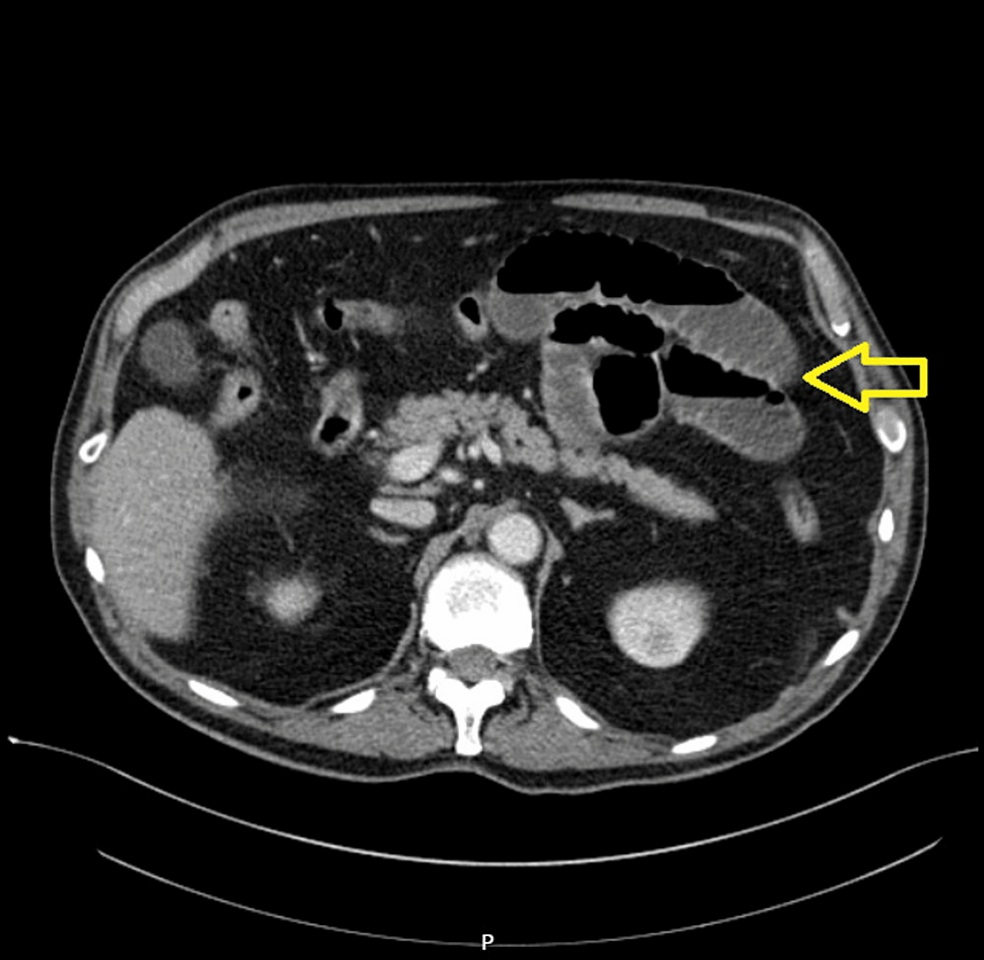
CT scan after gastrografin administration revealed distended small bowel loops with an air-fluid level inside.
